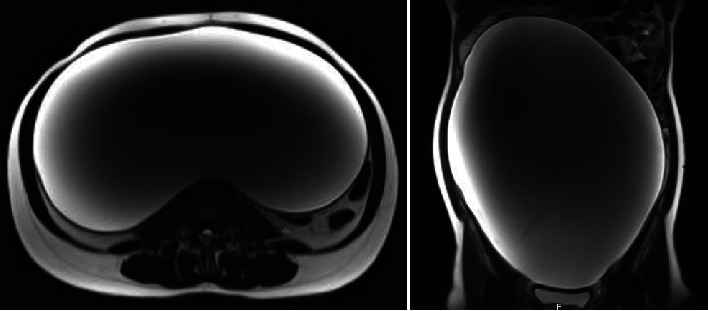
Pelvic magnetic resonance imaging showing a large well defined pelviabdominal cystic lesion.
